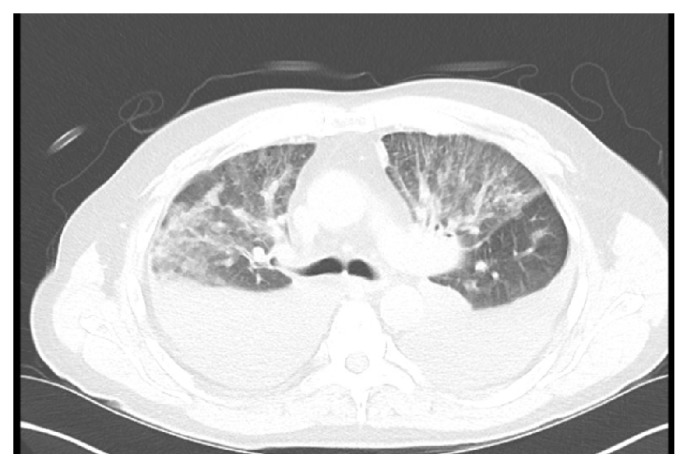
CT of the chest without contrast shows prominent parenchymal ground-glass changes. Large bilateral pleural effusions and moderate pericardial effusion.
